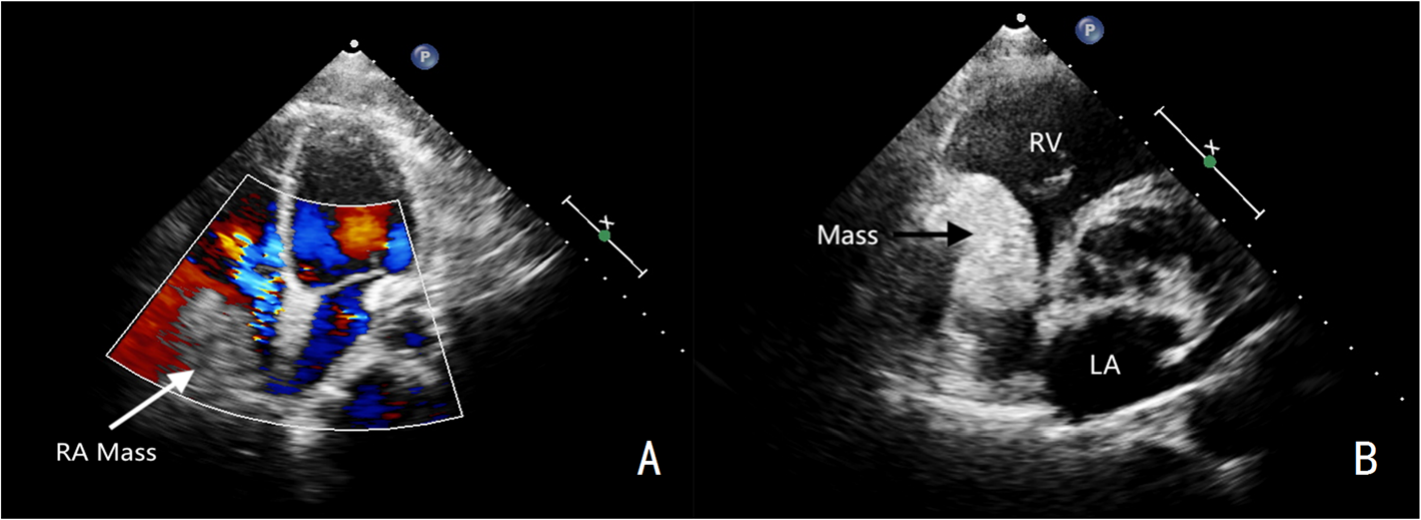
a The apical four chamber view demonstrates the right atrial mass (arrow), there is no blood flow signal within the mass. b During diastolic period, the mass (arrow) moves toward the tricuspid valve orifice, but does not enter the right ventricle. LA, left atrium; RV, right ventricle
